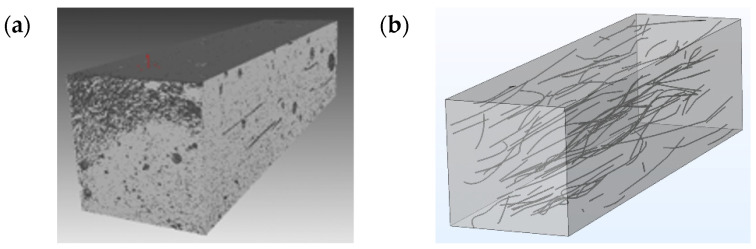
Representative volume element of size 950 μm × 950 μm × 3670 μm: (a) from μ-CT data, (b) reconstructed in COMSOL Multiphysics^®^. The fibers of the RVE were extracted from μ-CT data and imported into COMSOL Multiphysics^®^ via LiveLink™ [34].
